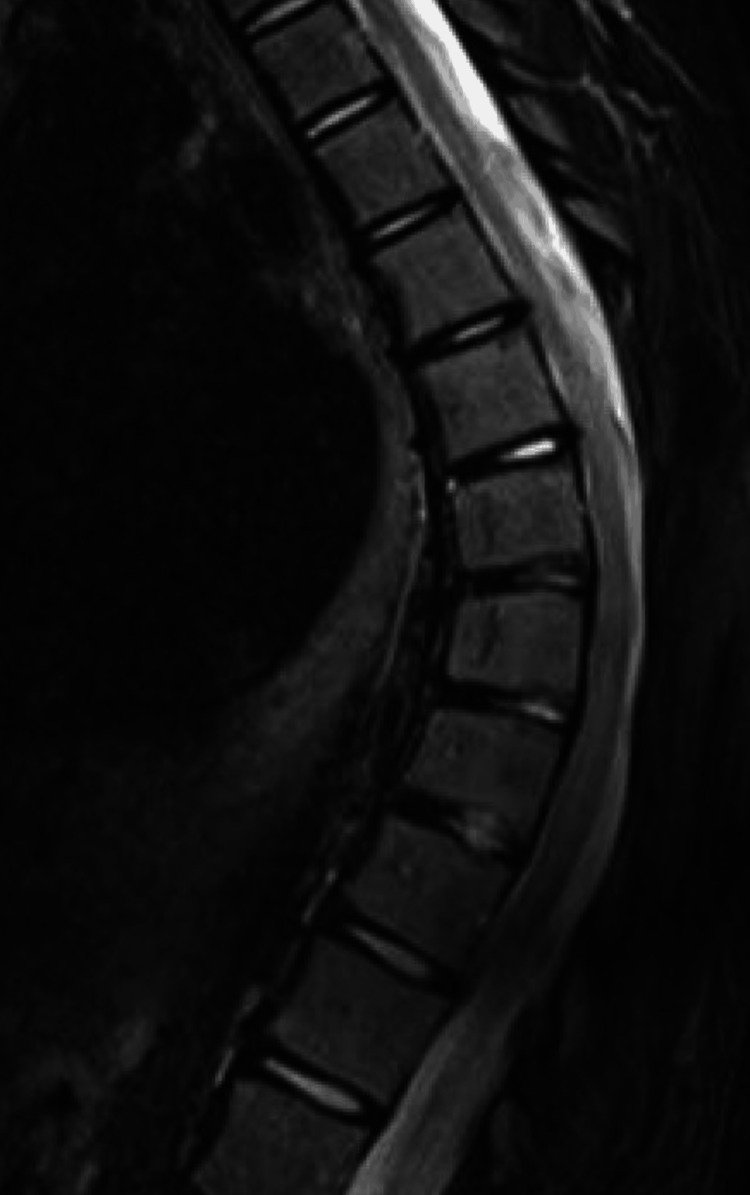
Postoperative sagittal T2-weighted MRI of the thoracic spine showing resolution of the herniation and absence of persistent dural defects or epidural fluid collections. The previously swollen segment of the cord demonstrates normalization.
